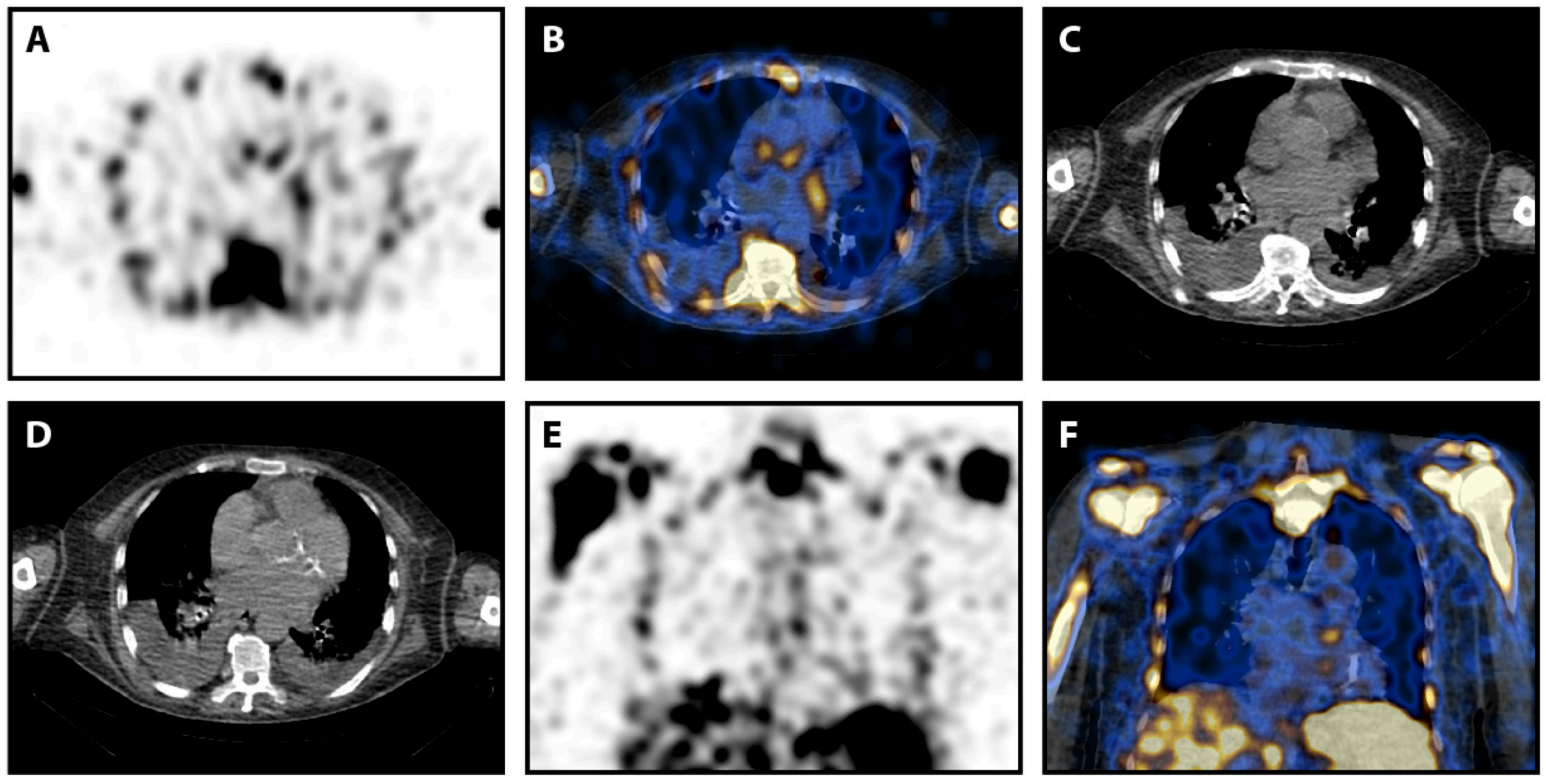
^99m^Tc-Besilesomab-SPECT/CT in a patient with suspected prosthetic valve infection and intra-observer difference. Images of a 78-years old male patient with suspected prosthetic valve infection. Blood cultures detected *Enterococcus faecalis* and TEE was negative. SPECT showed a trifocal uptake at the aortic valve level (A). This focal uptake was localized slightly cranial of the prosthetic valve in fused images (B). (C) Non-fused CT image at the same level as (B). (D) Non-fused CT image of the prosthetic valve caudal of (D). Focal uptake was also visible in coronal view (E,F). Observer 1 scored the focal uptake “2” while observer 2 scored it “1.” The patient had a fever above 38°C for 4 weeks. Clinical inspection detected Osler's nodes on the hands. Endocarditis was clinically confirmed according to Duke criteria.
